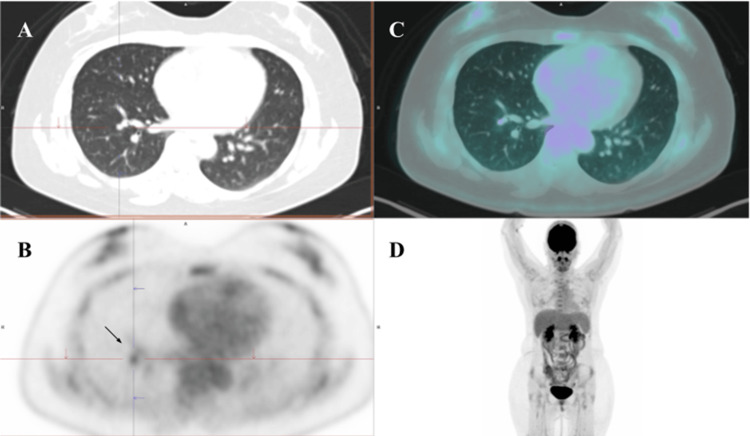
A) Axial CT (lung window): pulmonary lesion in the anterior basal segment of the right lower lobe. B) Axial PET: hypermetabolic focus in the anterior basal segment of the right lower lobe. C) Axial fused PET/CT image. D) Whole-body MIP image from FDG PET/CT. CT: computed tomography; PET: positron emission tomography; MIP: maximum intensity projection; FDG: fluorodeoxyglucose
